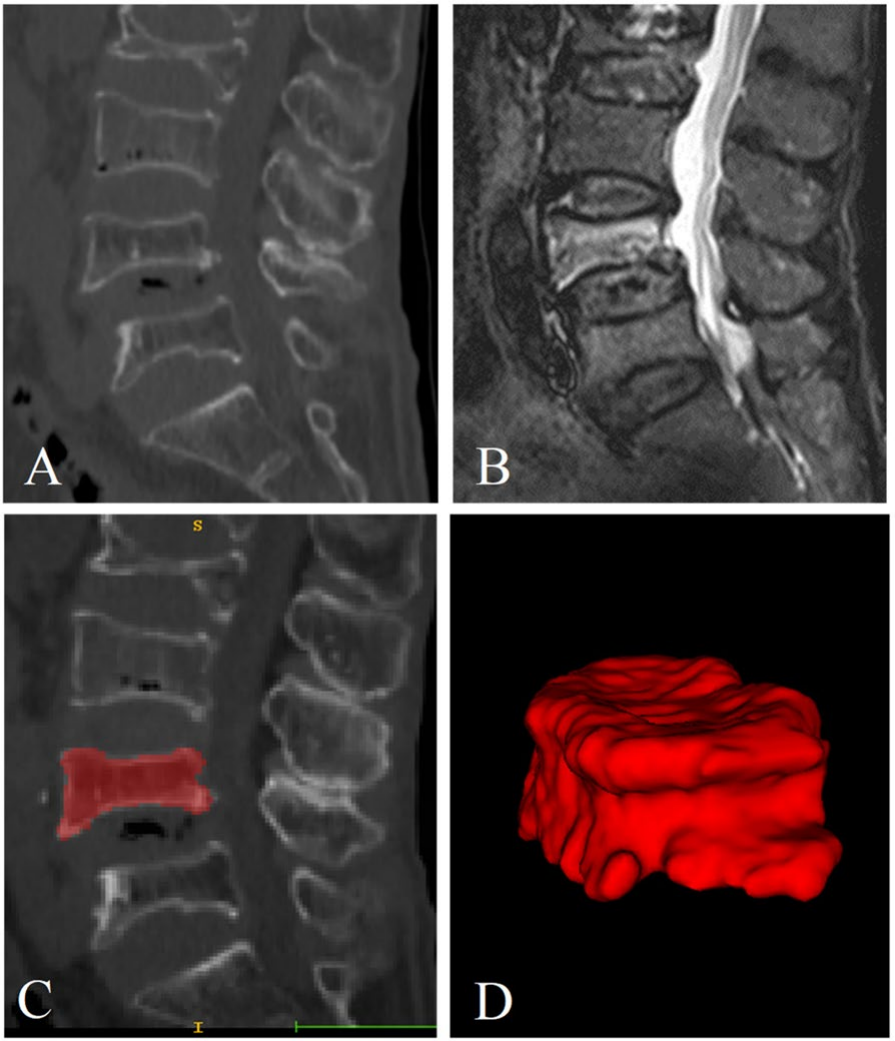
Segmentation of a fractured vertebral body for radiomic analysis in an 82-year-old woman with an acute compression fracture. A Compression fracture of L4 on sagittal non-contrast-enhanced spine CT images. B Hyperintense on sagittal T2 weighted fat-suppressed imaging of acute vertebral fracture. C ROI on sagittal CT images. D Three-dimensional volume meshes
